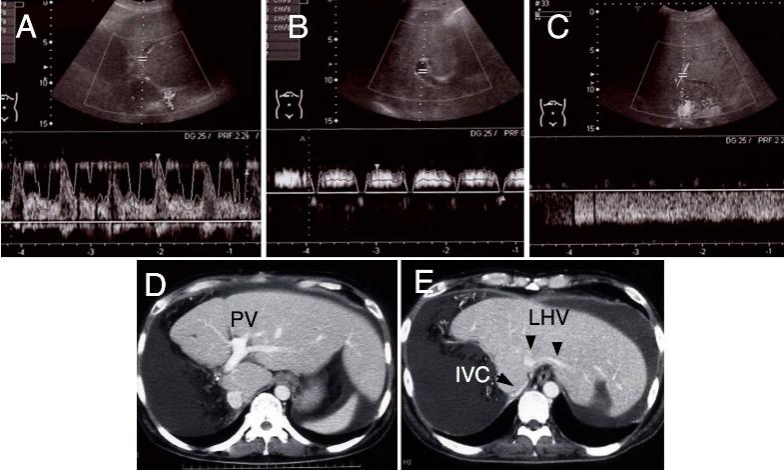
Spectral Doppler ultrasound study and contrast-enhanced computed tomography (CT) images at 7 months after surgery.
